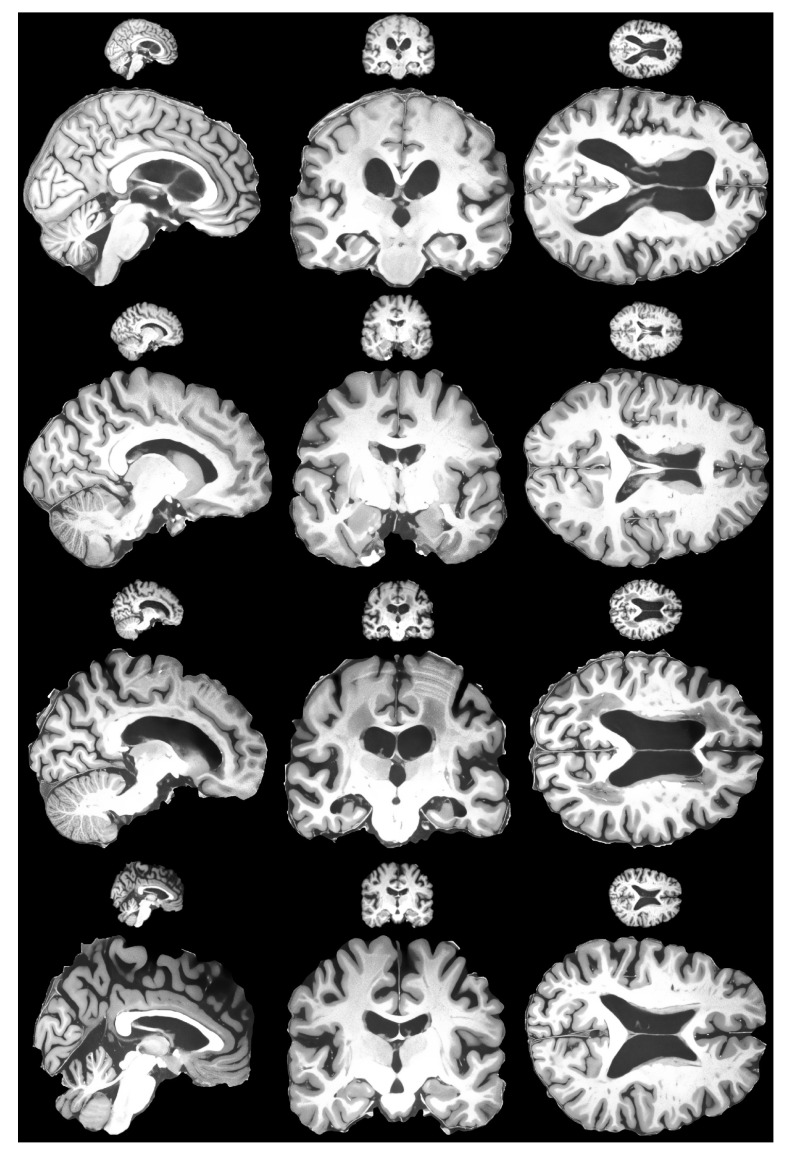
Subjective comparison of HR-MRI-GAN model results with OASIS 4 dataset. Figure shows four different patients’ brain scan slices for each plane.
